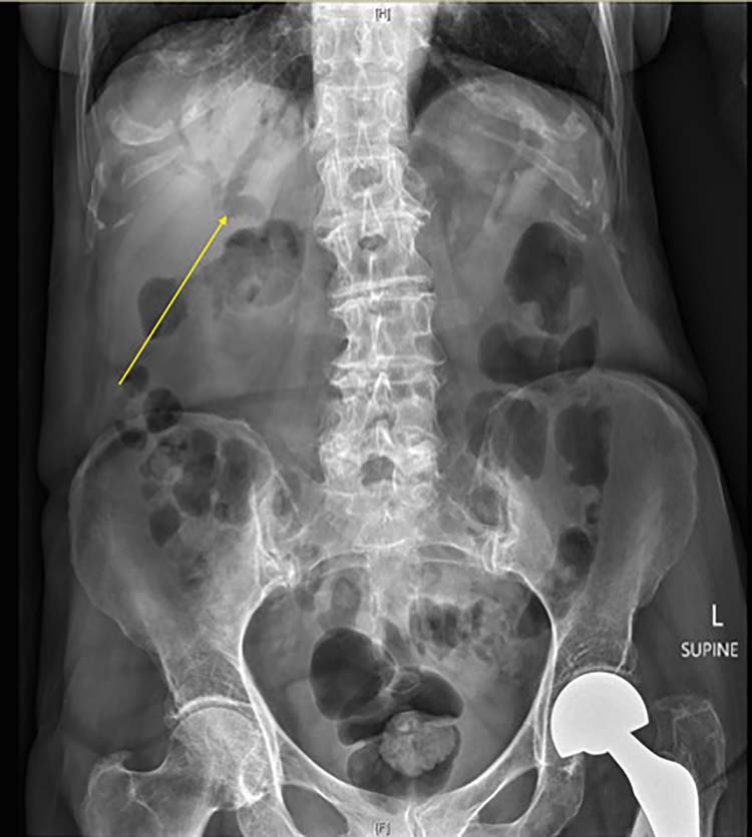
Abdominal X-ray with arrow demonstrating pneumobilia.
